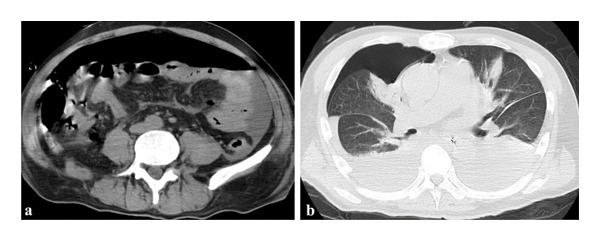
Computed tomography findings after the fourth direct endoscopic necrosectomy. (a) Abdominal computed tomography showing a large amount of free intraperitoneal air. (b) Chest computed tomography demonstrating right pneumothorax.
